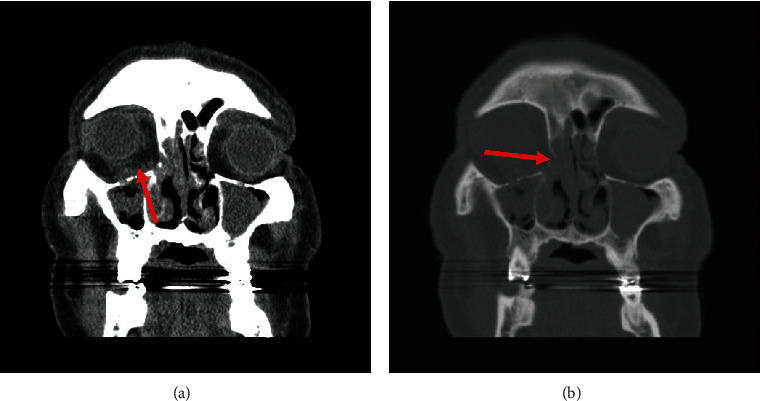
Coronal paranasal CT images without contrast administration. Soft tissue window images: (a) area of increased density in the inferior aspect of right orbit suggestive of orbital inflammation. Mucosal thickening in the right maxillary sinus and a retention cyst in the Lt maxillary sinus was also demonstrated. Bone window image: (b) thinning in the medial aspect of the inferior orbital wall at the right side, suggestive of a small bony erosion (compared to the Lt one).
